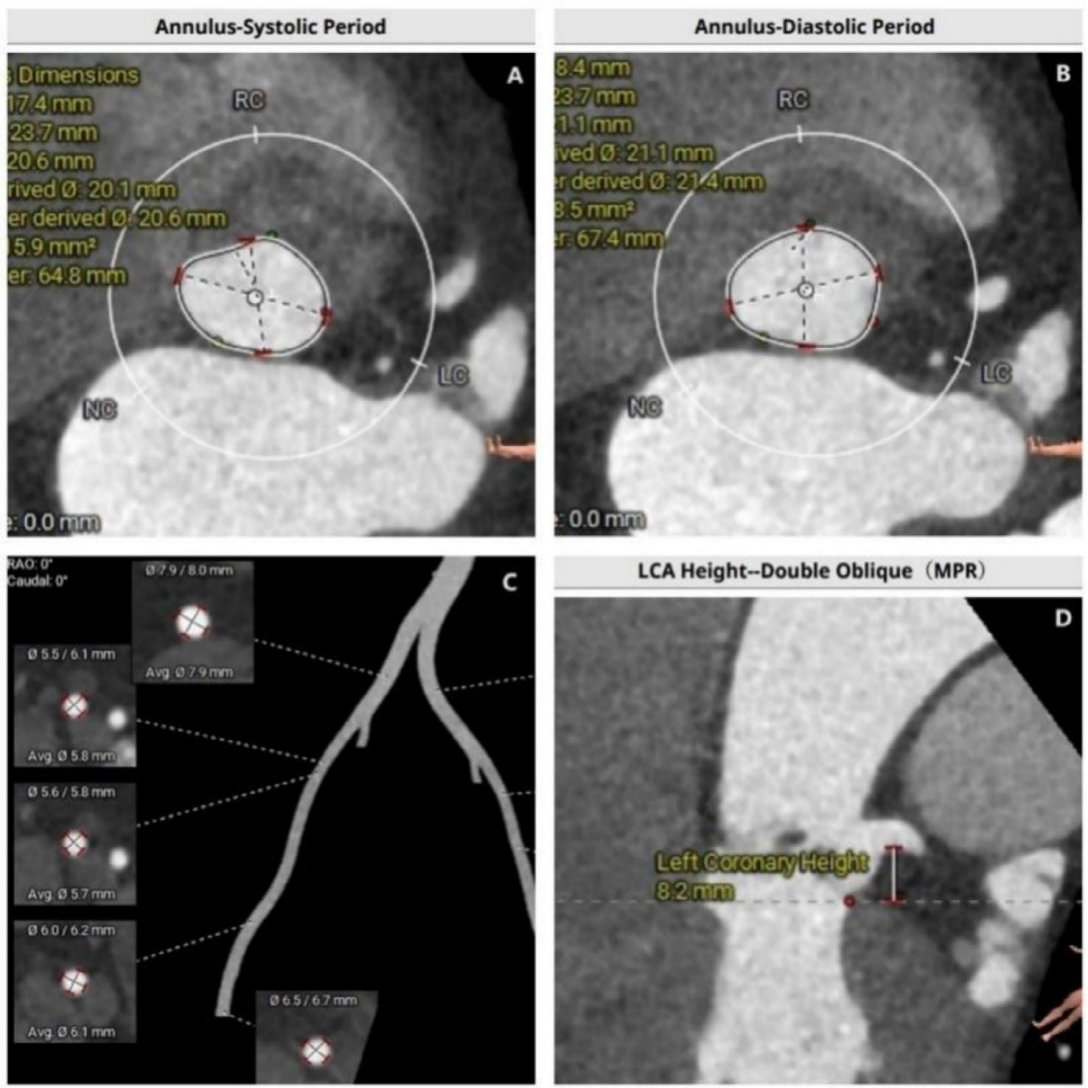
The assessment by computed tomography angiography (CTA). (A) Annulus‐Systolic Period (20.6 mm). (B) Annulus‐Diastolic Period (21.4 mm). (C) The minimum (narrowest) lumen diameter (5.7 mm). (D) Left Coronary Height (8.2 mm).
